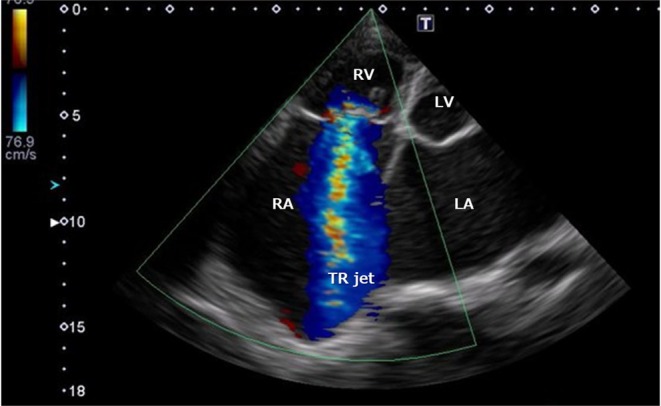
Transthoracic echocardiography before implantation showing a severe TR jet directed toward the septal side of RA. Transthoracic echocardiography demonstrated severe TR with a high‐velocity jet directed toward the septal side of markedly dilated RA, suggesting significant hemodynamic stress on the septal wall prior to implantation. LA, left atrium; LV, left ventricle; RA, right atrium; RV, right ventricle; TR, tricuspid regurgitation.
